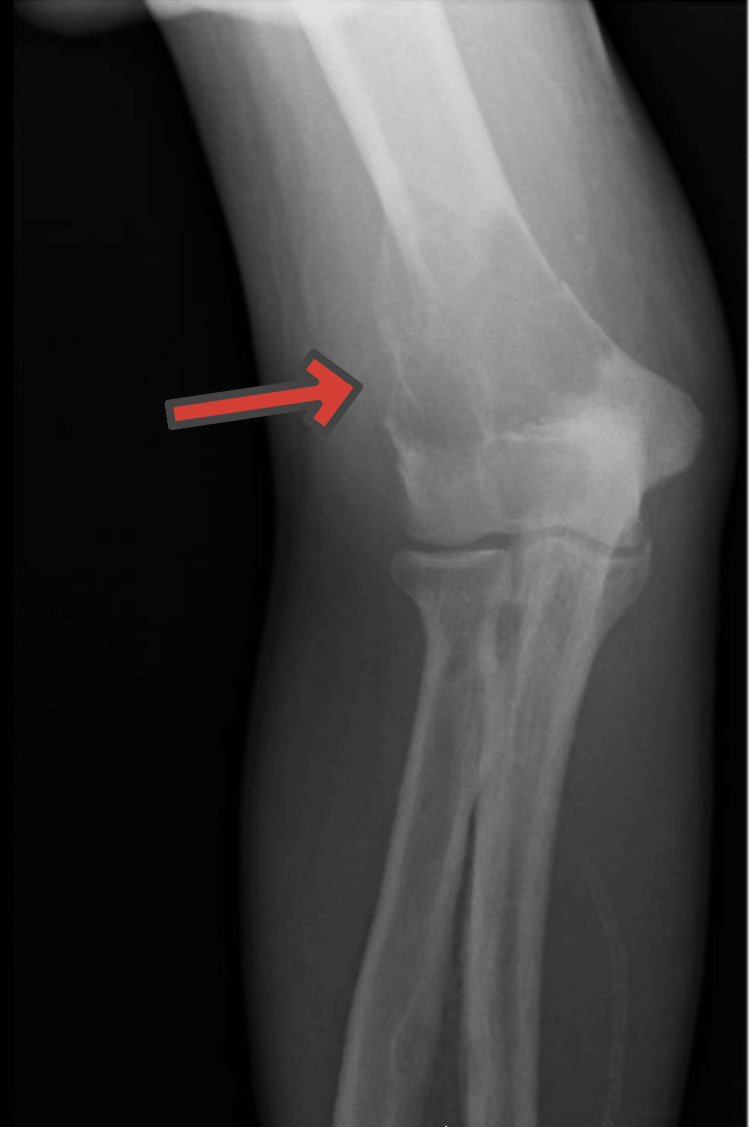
Two-view right elbow X-ray: 5 cm destructive process of the distal meta-diaphysis of the humerus with involvement of the entire diameter and mildly displaced pathologic fracture, cortical-based focus with peripheral sclerosis at the proximal diaphysis of the ulna, olecranon enthesopathy, soft tissue edema, and advanced vascular complication (arrow)
